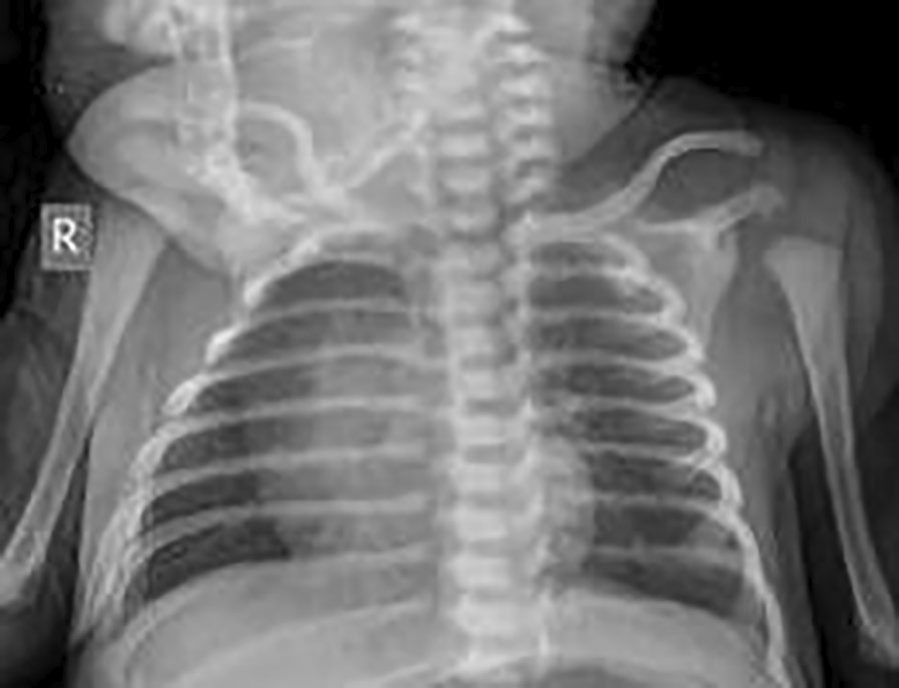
Anteroposterior chest X-ray view of term newborn showing no abnormal opacity in either lung and overall normal lungs
